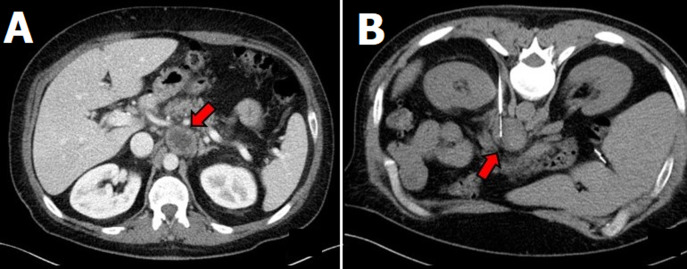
A) computed tomography (CT) abdominal scan revealing a 4cm nonspecific retroperitoneal, preaortic lesion, between celiac trunk and superior mesenteric artery; B) CT-guided biopsy of the retroperitoneal mass
